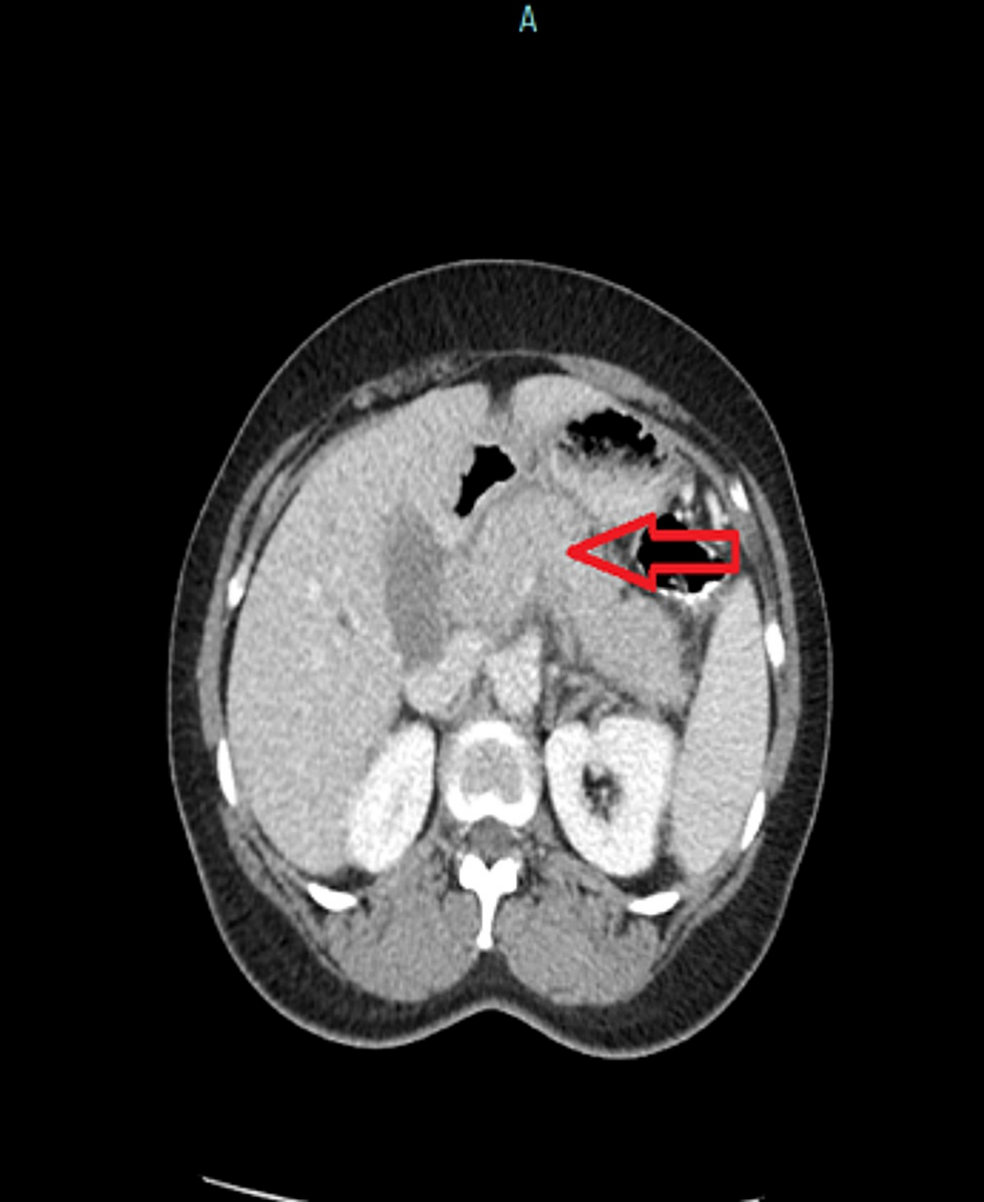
Pre-steroid treatment CT of the abdomen: pancreatic head and uncinate process appear enlarged, although no discrete enhancing pancreatic mass was identified (red arrow). CT: computed tomography
